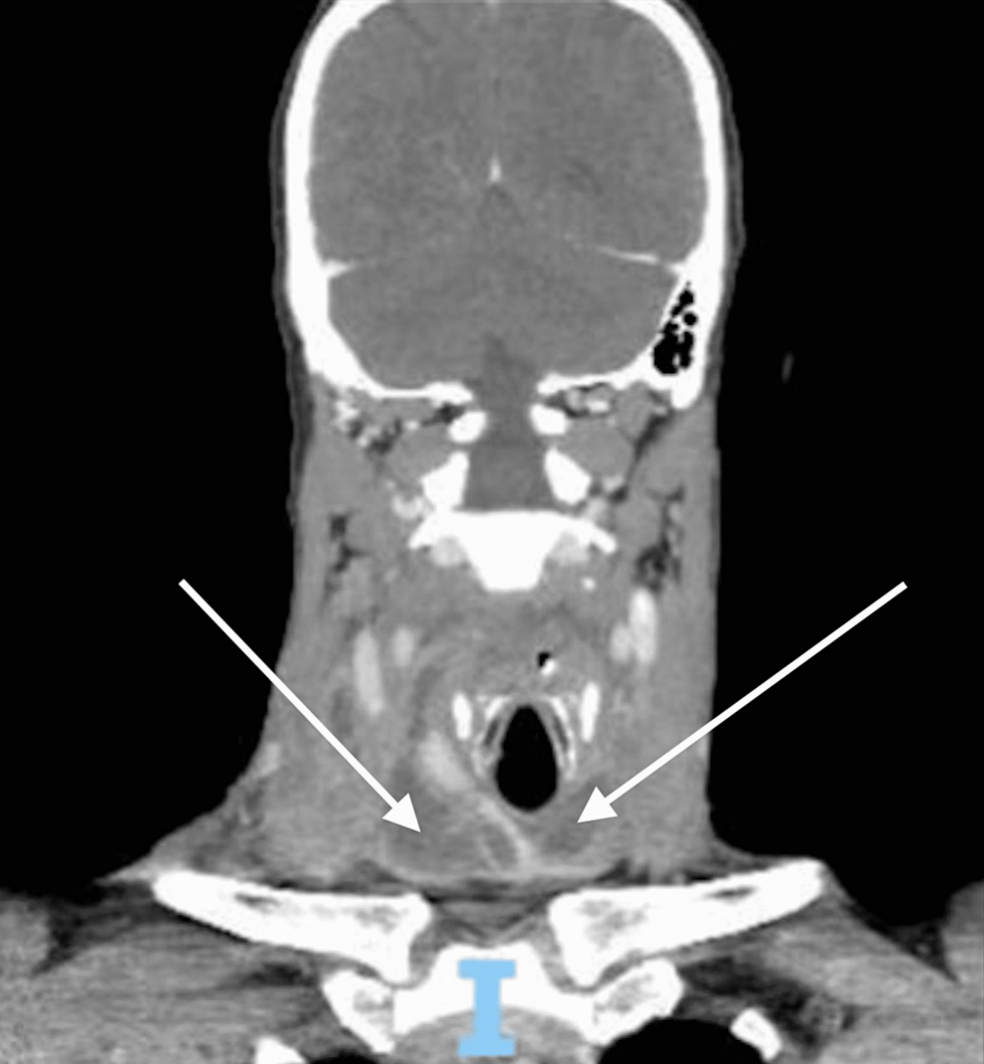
Coronal view of the CT scan on hospital day 5 White arrows represent fluid collection from descending infection.
